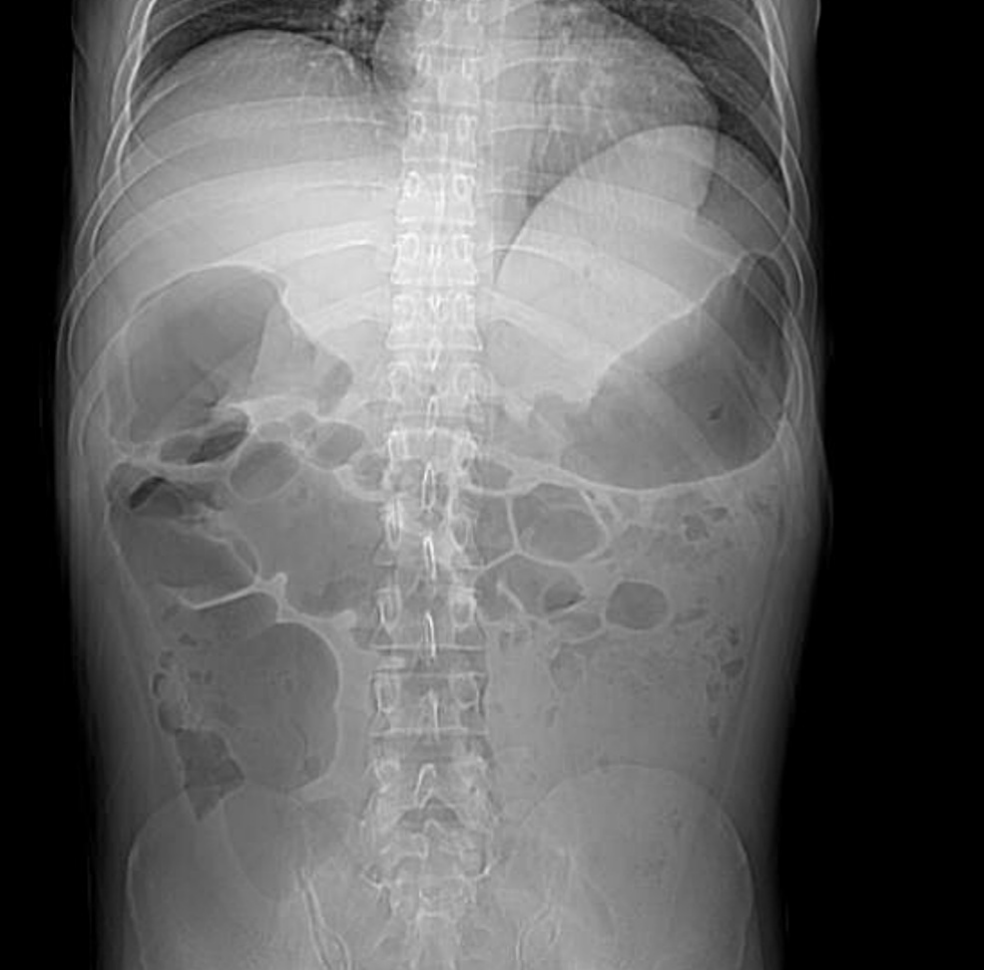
Follow up CT scan of the abdomen and pelvis showing significantly decompressed colon.
